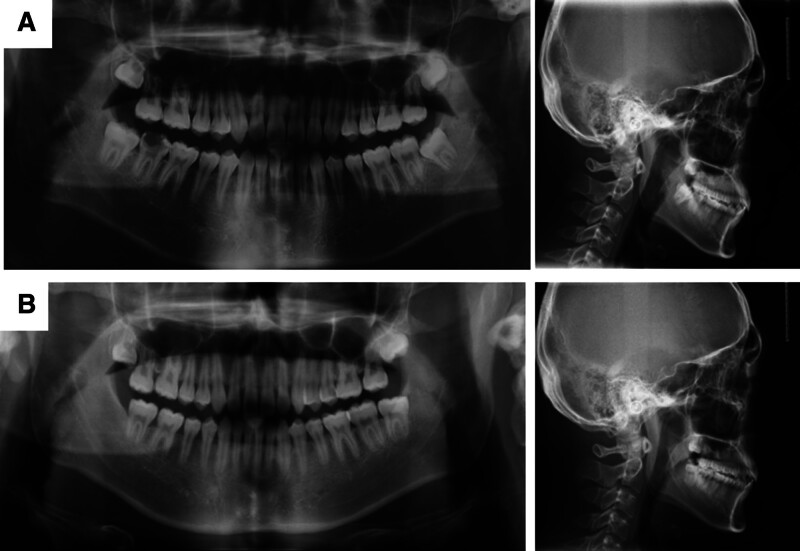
Pretreatment and posttreatment panoramic radiograph and lateral cephalogram.
